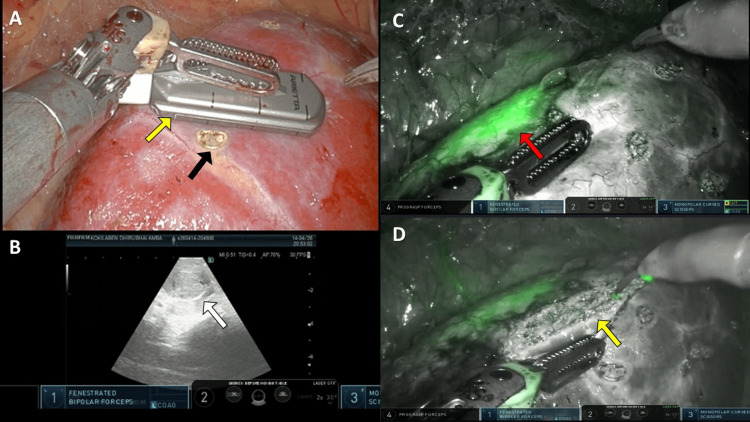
Intraoperative ultrasound and indocyanine green (ICG) fluorescence A) Intra-operative ultrasound probe (marked with yellow arrow) used for surface marking of the tumor (depicted with black arrow) using robotic scissors; B) Corresponding ultrasound image depicting the circumscribed tumor outline (shown with white arrow); C) ICG fluorescence (perfused area depicted with red arrow) used after clamping the anterior division of the right renal artery; helped to demarcate territorial distribution of arterial divisions; D) Incision being taken on the kidney, along Brodel's avascular plane (depicted by the yellow arrow)
